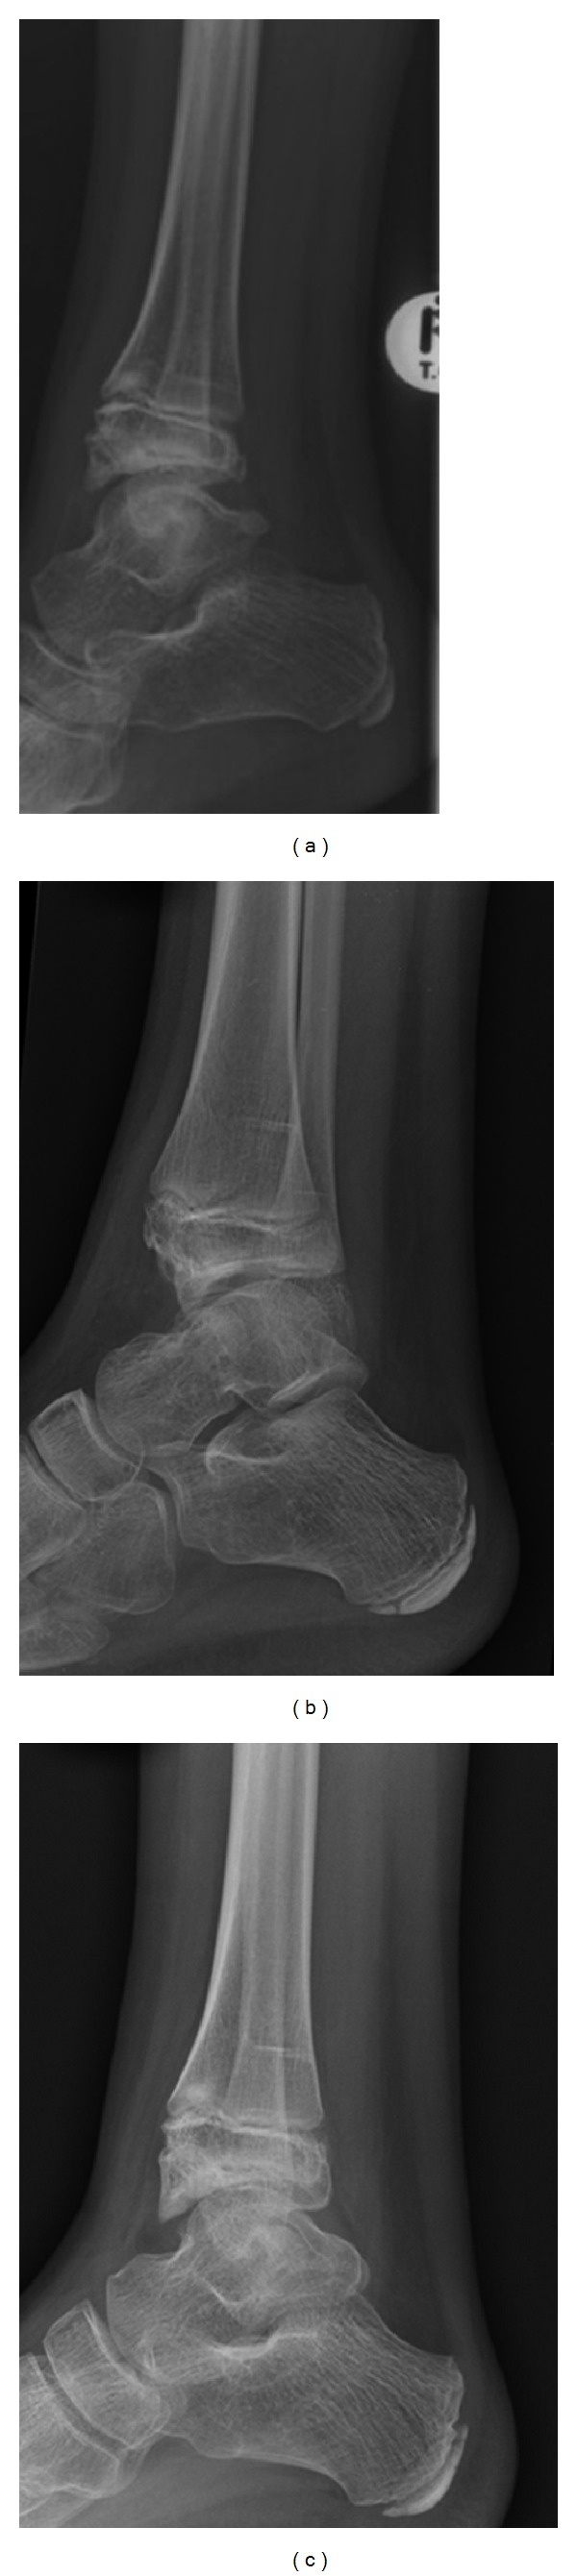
Serial X-rays: (a) preoperative in 2005, (b) postoperative in 2008, and (c) last followup in 2013, showing the lesion before and after its removal and the regrowth at the distal tibia.
